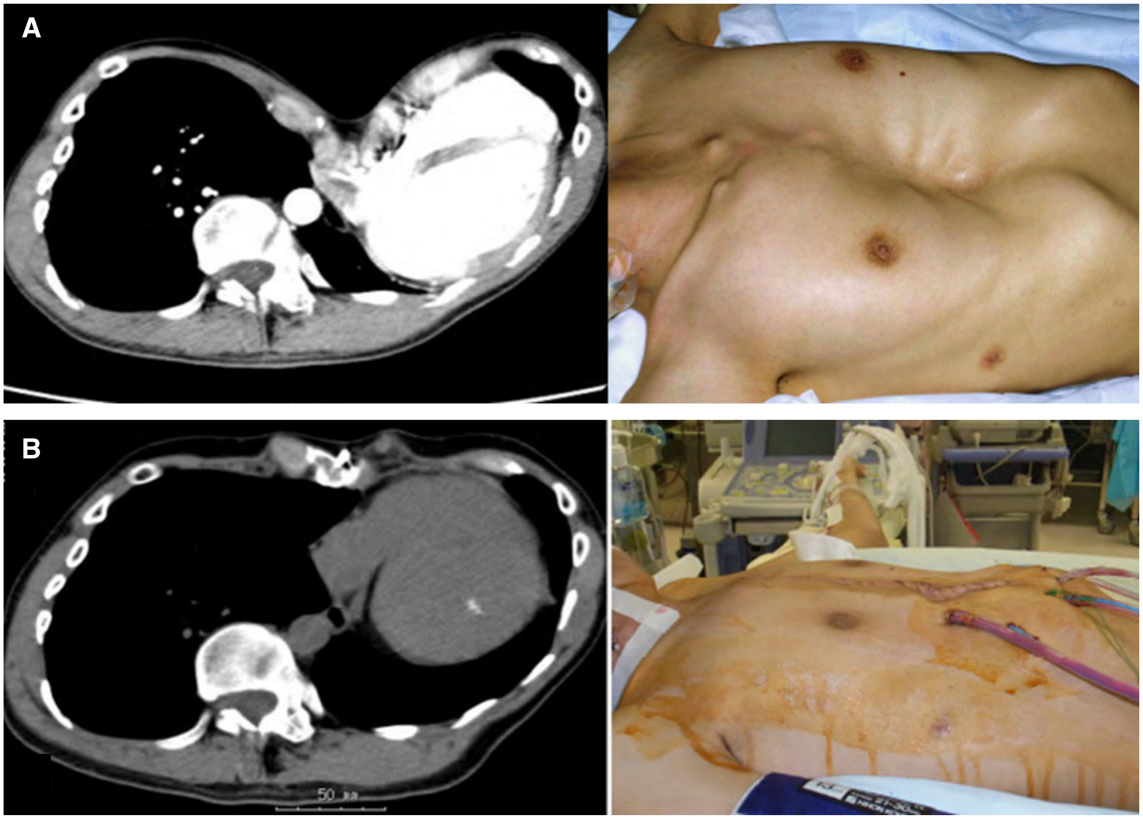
Computed tomography and picture (A) before and (B) after the surgical procedure. The morphology of the chest wall improved both visually and on computed tomography images before and after the surgery.
